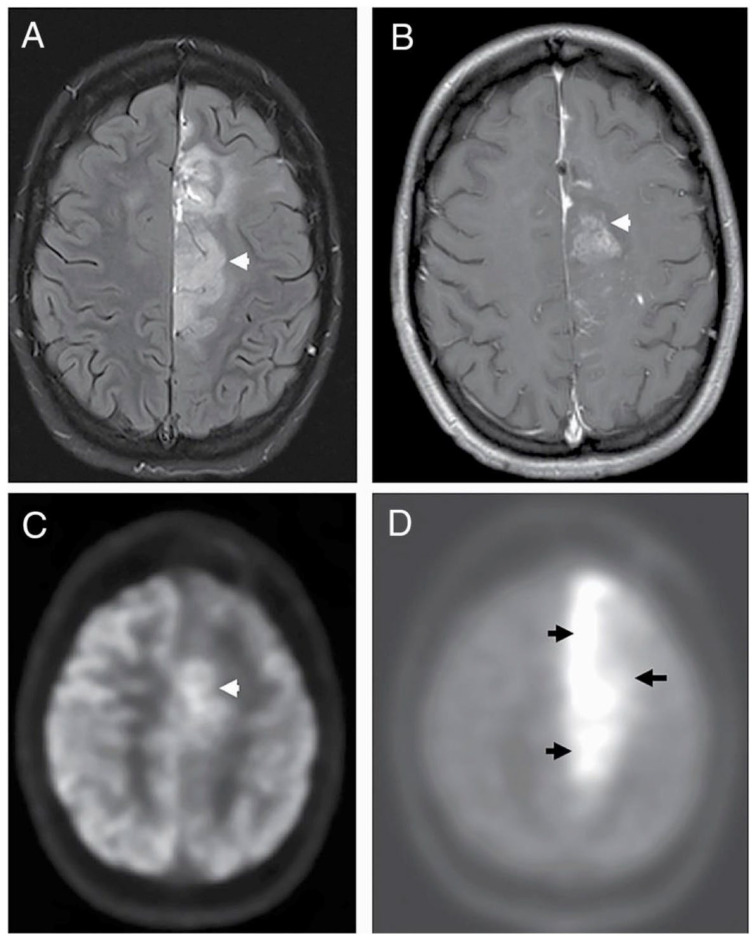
Metabolic imaging of glioblastoma at recurrence. (A) Axial T2 FLAIR images through the level of the centrum semiovale demonstrate a large, ill-defined, heterogenous tumor involving the body of the left cingulate gyrus that demonstrates patchy enhancement on the post-contrast T1-weighted sequence. (arrowhead) (B) 18FDG PET (arrowhead showing tumor) (C) demonstrates hypermetabolism involving only the anterior aspect of the tumor (arrowhead), whereas the 11C-Methionine PET and (D) depict the entirety of the tumor more conspicuously. (arrowheads outlining tumor area) Reproduced with permission from [25] © The authors 2022.
